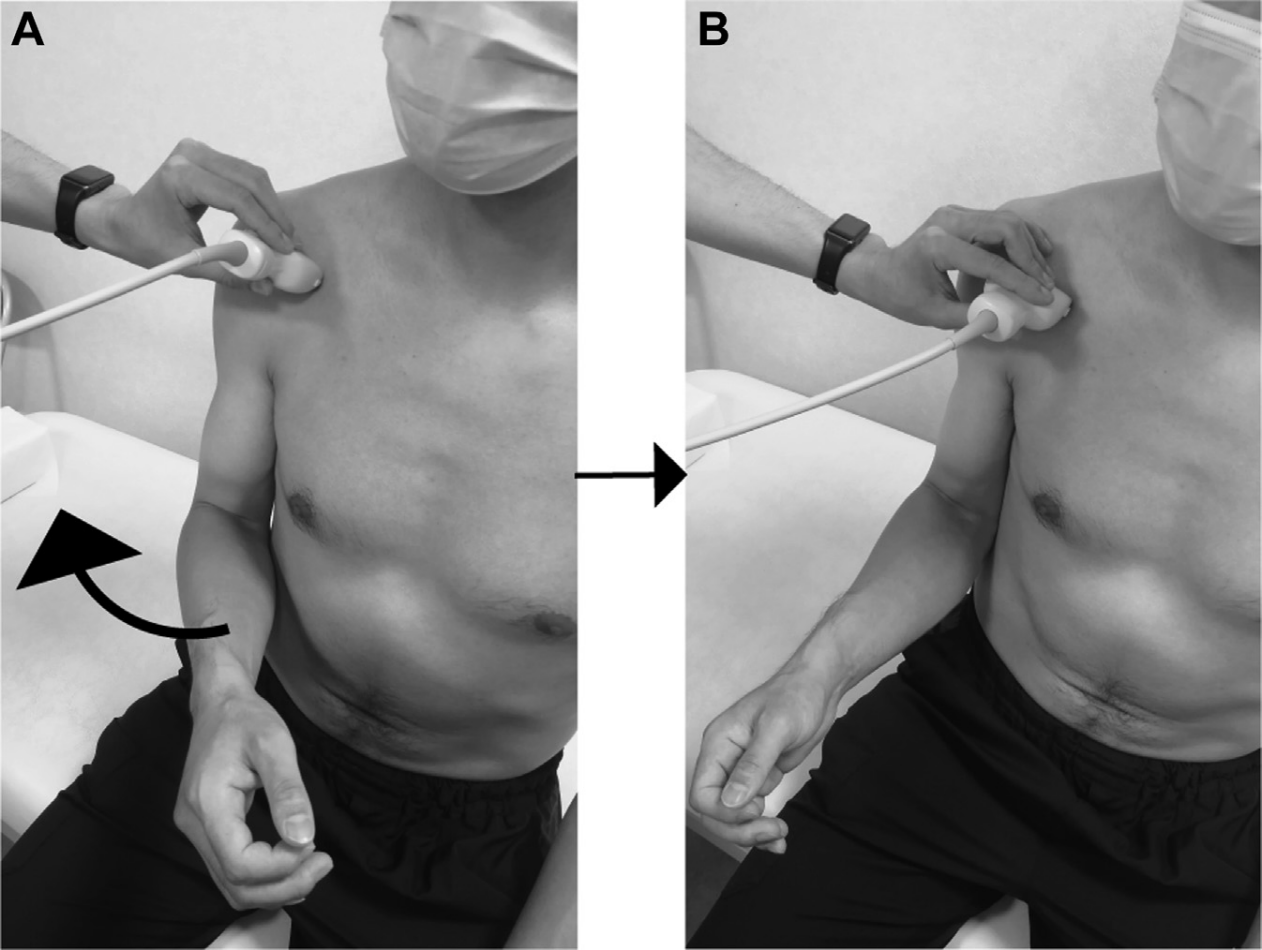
Dynamic ultrasonography setting. (A): Initial position of the motion task, (B): Terminal position of the motion task.
